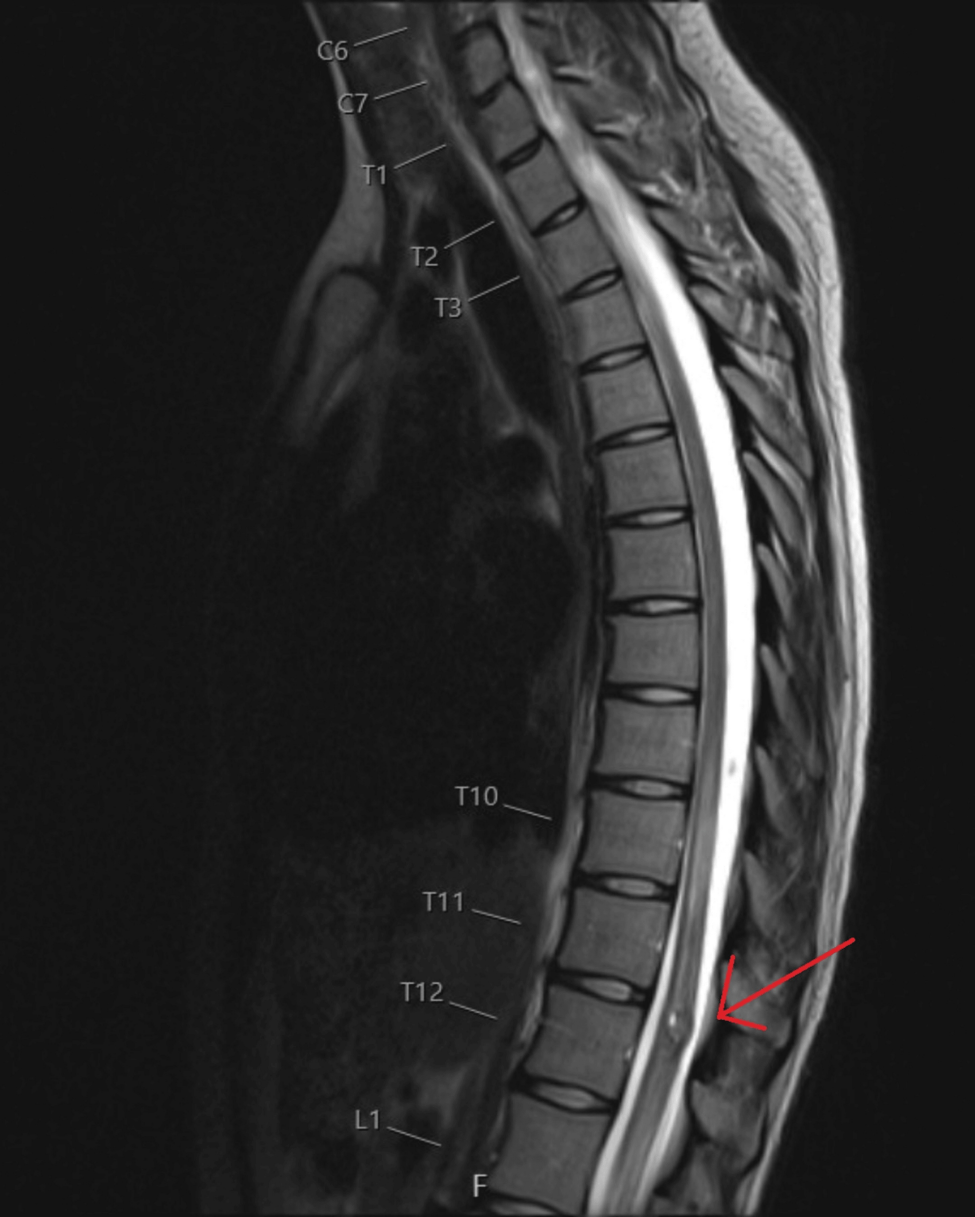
Sagittal T2-weighted MRI of the thoracolumbar spine showing a focal intramedullary lesion at the superior margin of T12 with a surrounding hypointense haemosiderin rim and associated cord oedema extending from T9 to the conus, consistent with a haemorrhagic spinal cavernoma Red arrow mark showing intramedullary iso-intense lesion consistent with a spinal cord cavernoma
